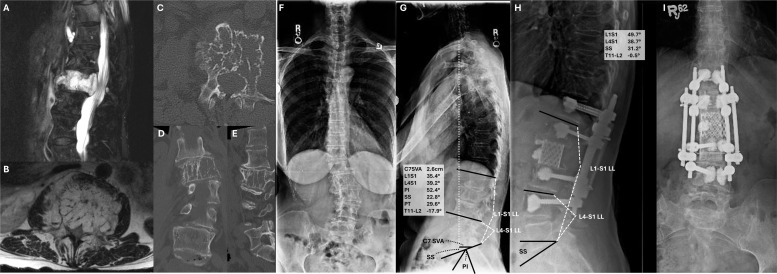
Case 1. Patient presented with mechanical low back pain and neurogenic pseudoclaudication. MR showed A) a T2-hyperintense, B) homogeneously contrast-enhancing lesion of the L2 body with focal collapse and bony retropulsion, causing spinal canal stenosis. C–E) CT demonstrated extensive osteolysis in all planes. F, G) Upright full-length scoliosis films demonstrated a mild levoscoliosis with relative preservation of sagittal balance but a mild reversal of lordosis at the thoracolumbar junction. H, I) Postoperative upright radiographs show reconstruction with a T12-L4 instrumented fusion with titanium cage reconstruction in the L2 corpectomy defect.
